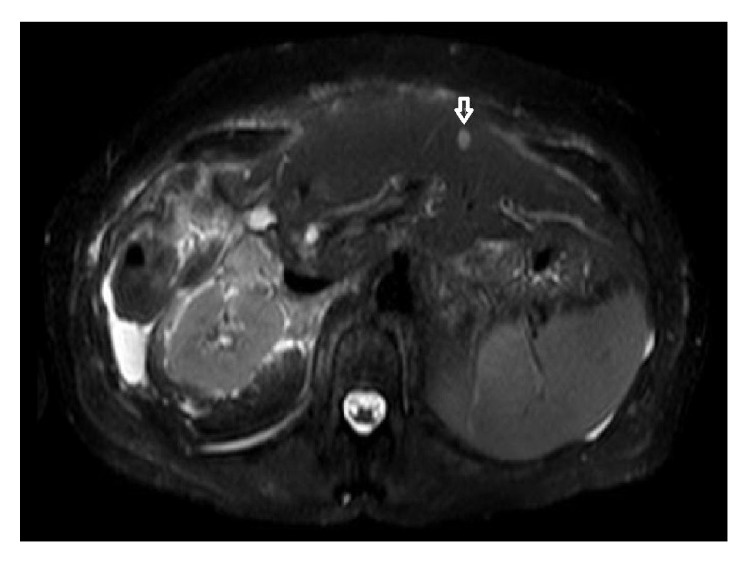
Postoperative follow-up MRI (small white arrow indicates new suspicious lesion).
